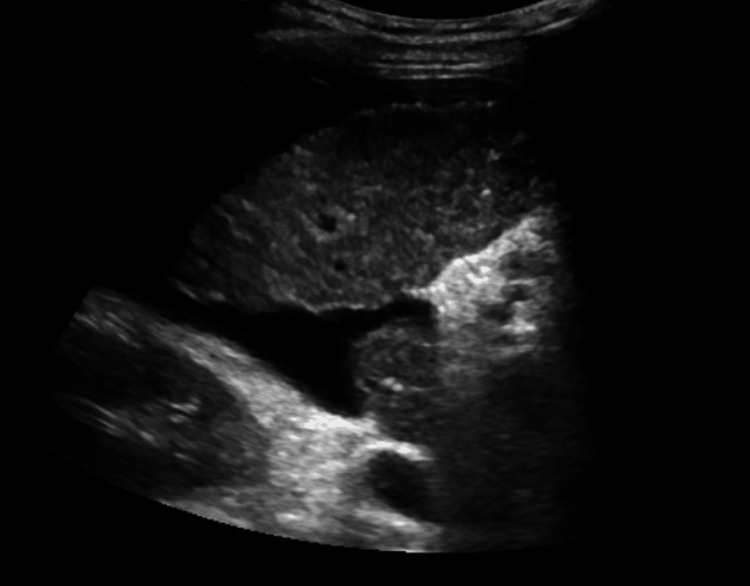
Abdominal ultrasound (transverse view of the right lobe) showing heterogeneous, coarsened hepatic parenchyma with a nodular contour consistent with cirrhosis.
